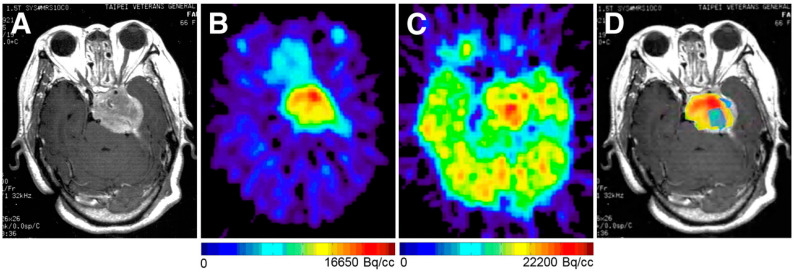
The figure demonstrates a patient with an anaplastic meningioma (Grade 3). (A) Contrast-enhanced MR image demonstrates a necrotic lesion in the middle fossa of the left skull base. (B,C) PET images reveal an increased uptake of both 1–11Cacetate (B) and 18F-FDG (C) in the tumor. (D) Co-registered PET imaging shows that the highest foci of 18F-FDG uptake (blue) coincide with areas of low 11C-acetate activity (yellow), with no overlay observed on the 11C-acetate hotspot (red). Adapted from Liu et al., 2010 [26].
